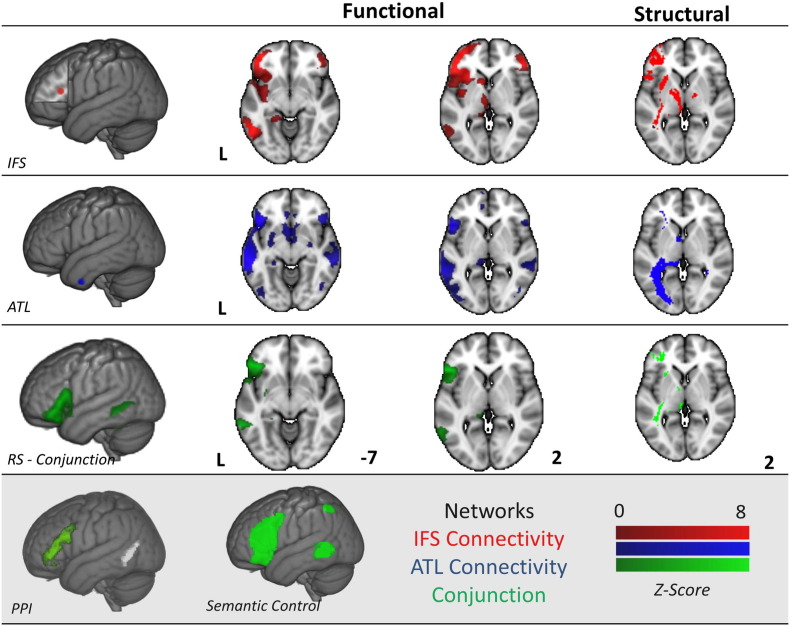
Resting state functional connectivity and structural connectivity (tractography) for functional peaks identified for hard semantic judgements in a key executive region (− 42 28 16, inferior frontal sulcus — IFS, in red) and easy semantic judgements in a region linked to semantic representation (− 48 2 − 38, anterior temporal lobe — ATL, in blue). The conjunction for the two connectivity patterns is displayed in green. All contrasts and conjunctions are cluster corrected to control for multiple comparisons (inclusion threshold z = 2.3, cluster significance = p < .05). The left-hand column shows the seed regions, columns 2 and 3 show resting state connectivity and white matter (WM) fibre tracts identified using diffusion MRI for each seed and their overlap are displayed on the right. We present the spatial maps from the Noonan et al. (2013) meta-analysis and from the prior PPI analysis to facilitate visual comparison of these three networks. Note the colour bar does not refer to the DTI Images which were corrected using randomise and are presented as fully saturated maps.
